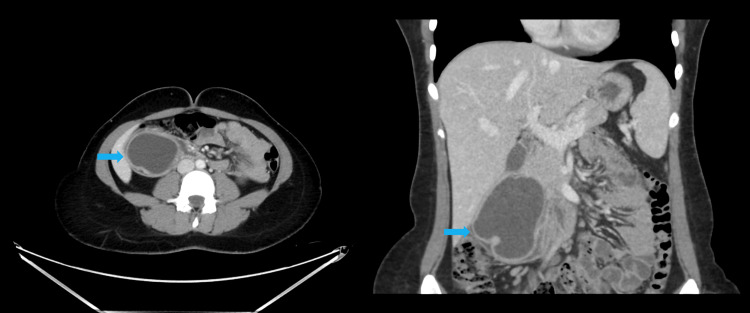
Axial (left) and coronal (right) portovenous phase of CT abdomen with IV contrast showing an 8.3 × 4.0 cm tubular cystic lesion (blue arrows) in the second part of the duodenum The change in the shape of the tubular structure is evident across three phases: portovenous, post-oral contrast, and lateral decubitus (Figures 1-3). These findings suggest a large intraluminal DD (blue arrows) in the second and third parts of the duodenum, without signs of cholecystitis, diverticulitis, or other complications. DD, duodenal diverticulosis
